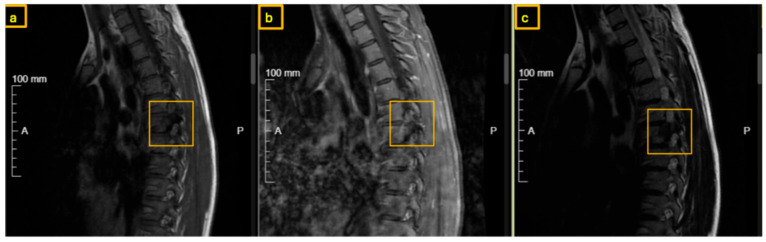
Postoperative MRI of the thoracic spine at 3-month follow-up. The yellow square indicates recurrence of the nidus at the root of the right T7 pedicle, with possible involvement of the vertebral body: (a) T1-weighted, (b) T1-weighted contrast-enhanced, and (c) T2-weighted sagittal views.
